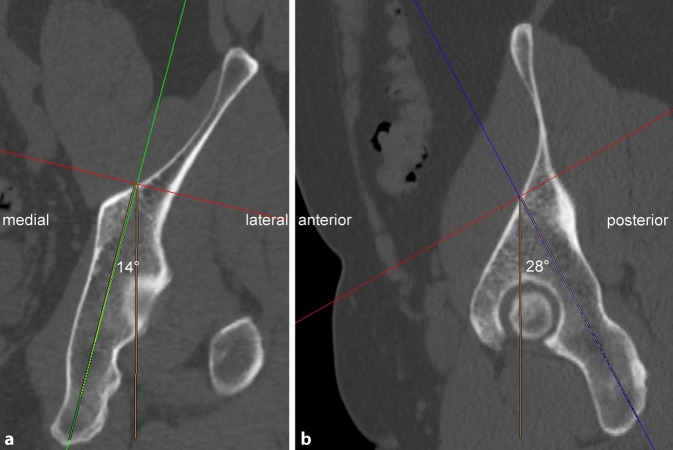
Preoperative assessment of the osseous corridor and ideal posterior column screw trajectory. We highly recommend to assess the ideal posterior column screw trajectory using the uninjured contralateral acetabulum because screw trajectory analysis is easier and more precise in absence of fracture lines and displacement. The uninjured contralateral acetabulum can be reliably used as a template for preoperative planning as the left and right posterior column anatomy (screw insertion angles, screw starting point and screw length) do not significantly differ within the same pelvis (see Results). In case of no or only minor displacement, however, preoperative planning may be also performed on the injured side. The starting point of the posterior column screw is located in the transition zone between the supraacetabular region and the iliac wing on the inner cortex of the iliac bone. The screw trajectory is oriented from cranial–anterior–lateral to caudal–posterior–medial (a, b). The ideal starting point and screw trajectory are assessed by using native axial CT images and a two-dimensional multiplanar software reconstruction tool. Therefore, the axes of coordinates are translated and the axes itself rotated to assess the ideal entry point and screw trajectory. In the present case, the medial angle of the ideal posterior column screw trajectory is 14° to the sagittal plane (a) with a maximum screw length of 130 mm from the starting point to the end point (cortex of the sciatic tuber). Furthermore, the posterior angle of this screw trajectory is 28° to the coronal plane (b) and obviously shows the same maximum screw length in this second reformation plane
